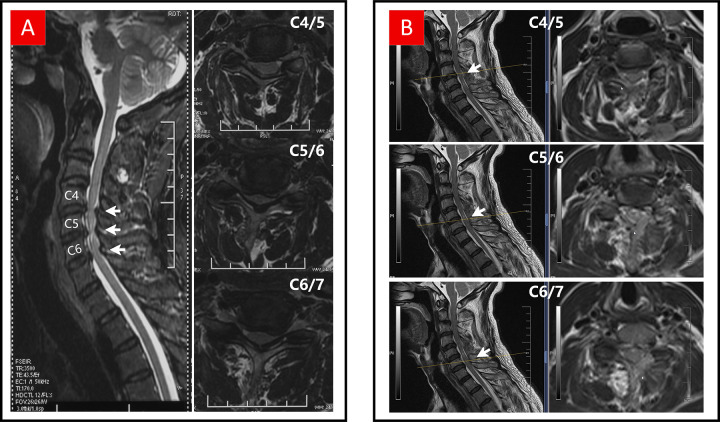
(A) Preoperative MRI of the patient showing spinal canal stenosis at C4/5, C5/6, and C6/7 levels. (B) Postoperative MRI following UBE posterior cervical decompression.
